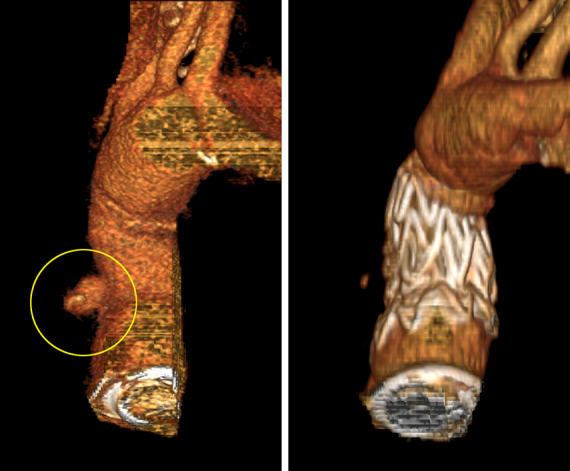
Reconstructed computed tomography image shows the pseudoaneurysm and its exclusion.
